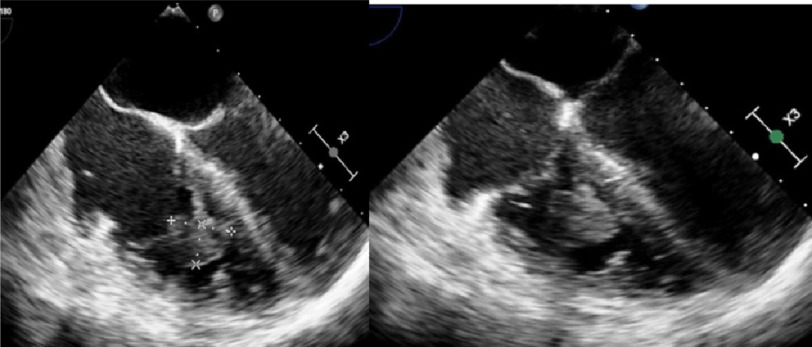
Repeat Transesophageal Echocardiography shows a large pedunculated, well-circumscribed mass in the tricuspid valve apparatus measuring from 2.1 cm to 1.8 cm. There was a small linear, mobile echo density visualized on the mass, which may represent a small thrombus.
